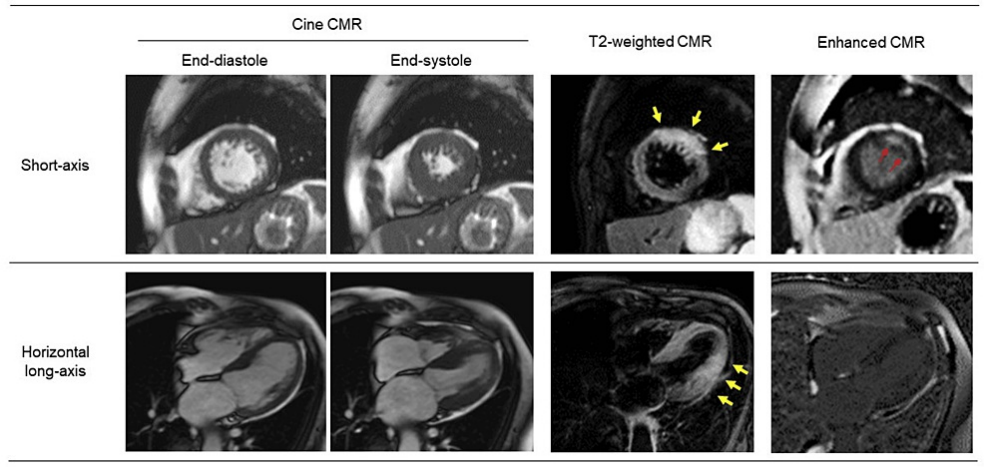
Cardiac magnetic resonance images Cine cardiac magnetic resonance (CMR) revealed no wall motion abnormalities including the area corresponding to the two branches. T2-weighted CMR revealed myocardial edema in the left ventricular anterolateral area (yellow arrows) with subendocardial late gadolinium enhancement (red arrows).
